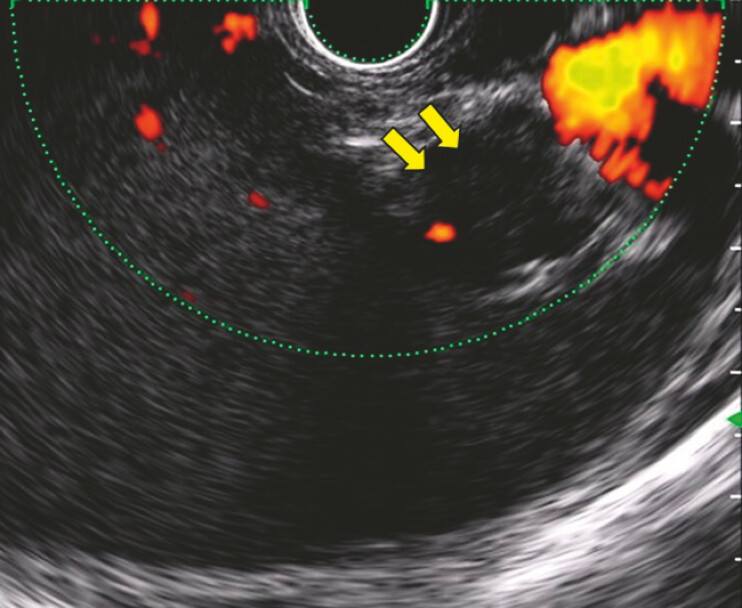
Endoscopic ultrasound revealed that the coils were placed correctly (yellow arrows) and the variceal flow had disappeared.
